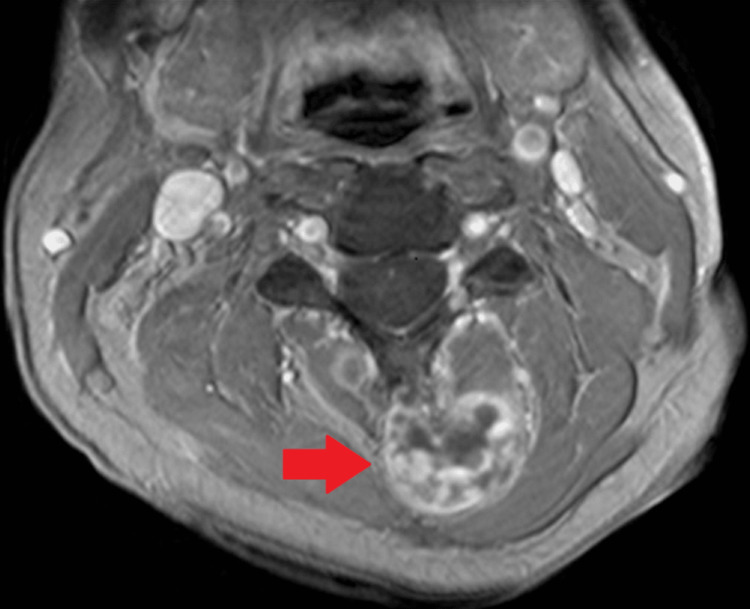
MRI of the cervical spine with contrast. Axial T1-weighted post-contrast image demonstrating heterogeneous enhancement of the tumor in the left lamina of C3 (red arrow).
